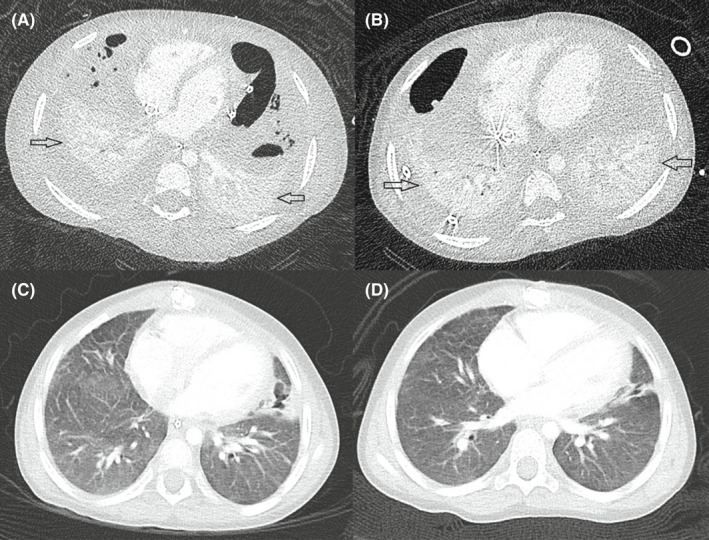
(A) CT Thorax done on Day 7 of extracorporeal membrane oxygenation (ECMO) shows bilateral collapse and consolidation (arrows). (B) CT Thorax on Day 37 of ECMO shows bilateral collapse and consolidation (arrows). (C and D) CT Thorax 2 months and 7 months post‐ECMO, respectively, showing significant improvement with minimal residual parenchymal changes
